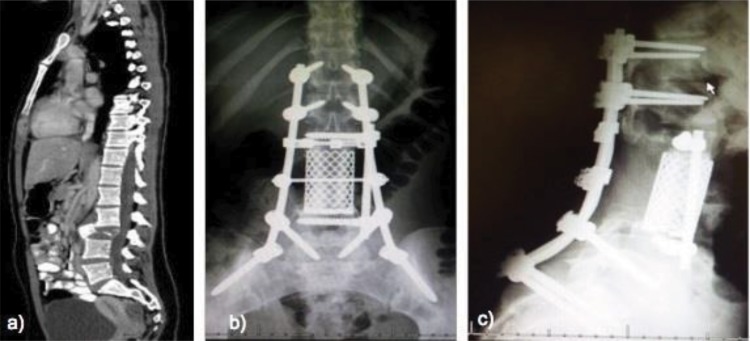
Computed tomography images of an 18-year-old patient with a giant cell tumor in L3. a) preoperative imaging b) and c) after en bloc vertebrectomy.
